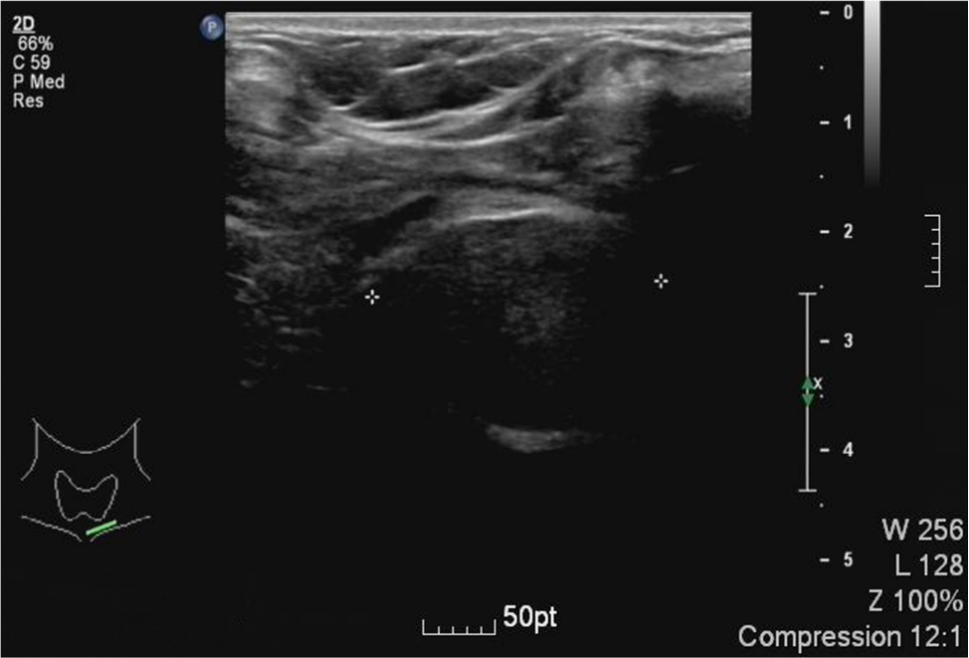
Ultrasound scan of a separate nodule under the left thyroid lobe. The ultrasound scan revealed a separate nodule under the left lobe.
